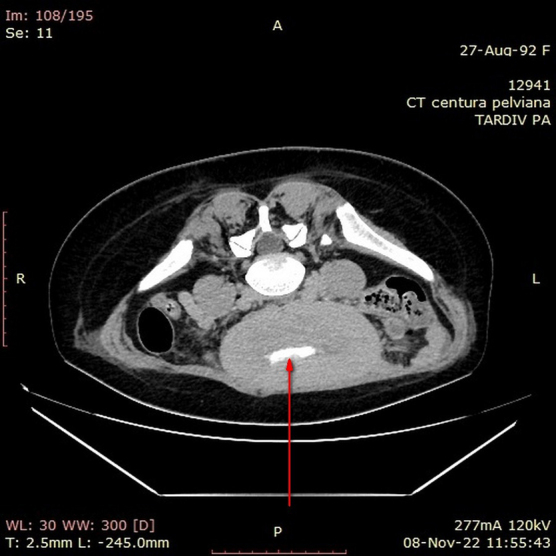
Contrast in the uterine cavity (CT, axial plane).
